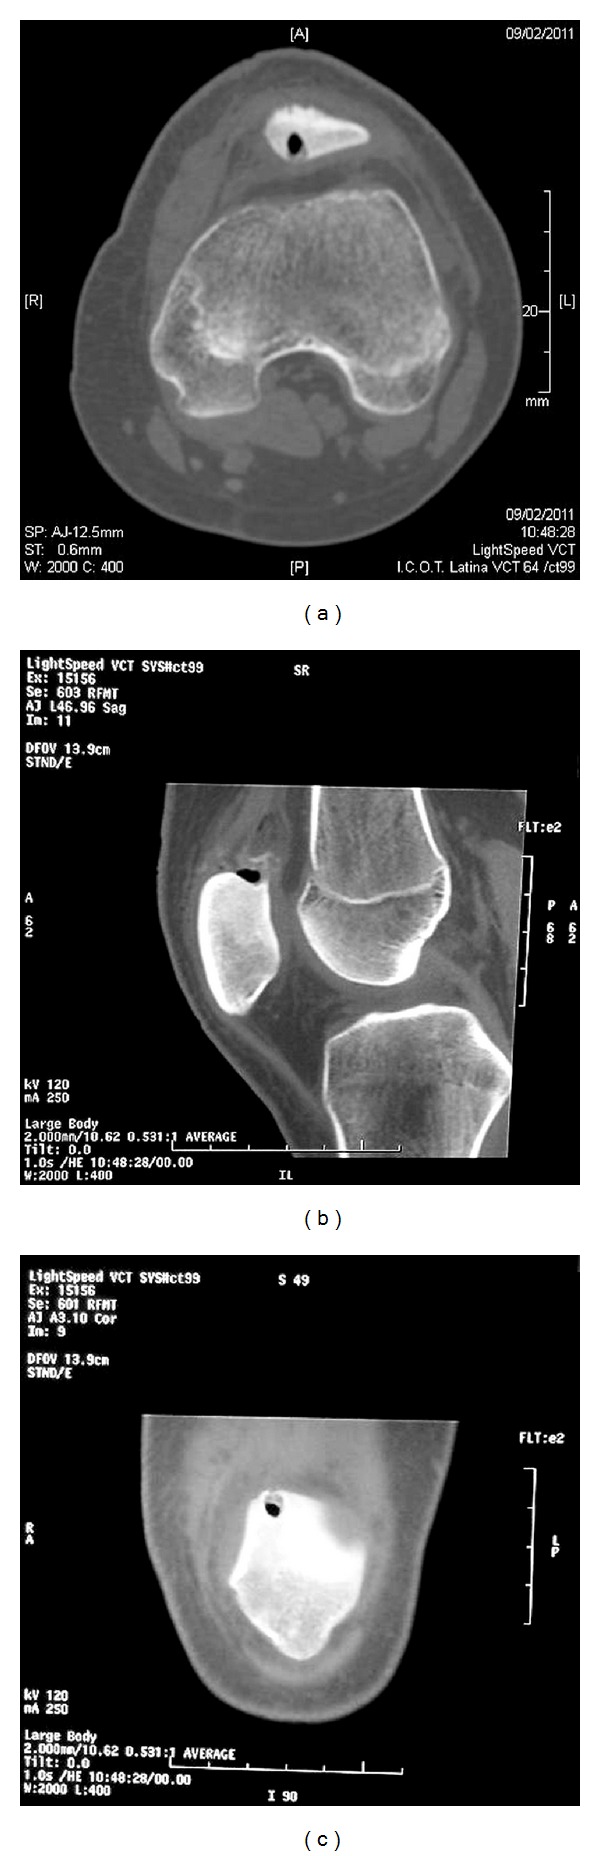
Postoperative CT scans confirm the disappearance of the nidus ((a) axial; (b) sagittal; (c) coronal view).
